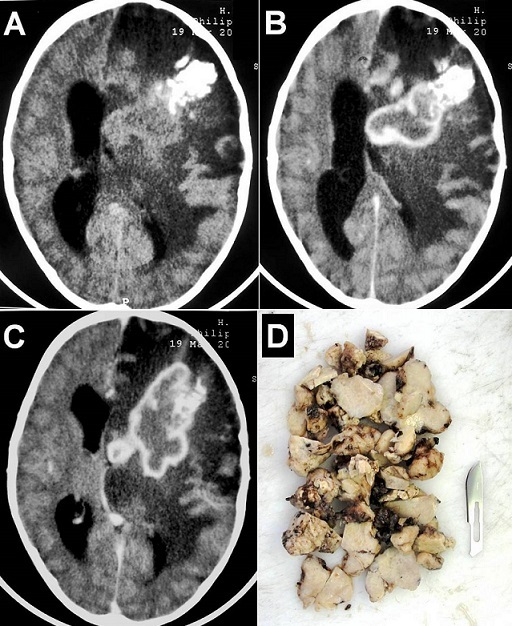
Brain computed tomography-scan before (A) and after contrast injection (B and C) showing a heterogeneous calcified large mass-like lesion in the left fronto-temporo-parietal region, irregular contrast enhancement and extensive perilesional edema with important mass effect. (D) External photograph of the large yellowish, firm, and relatively avascular tumor-like mass
